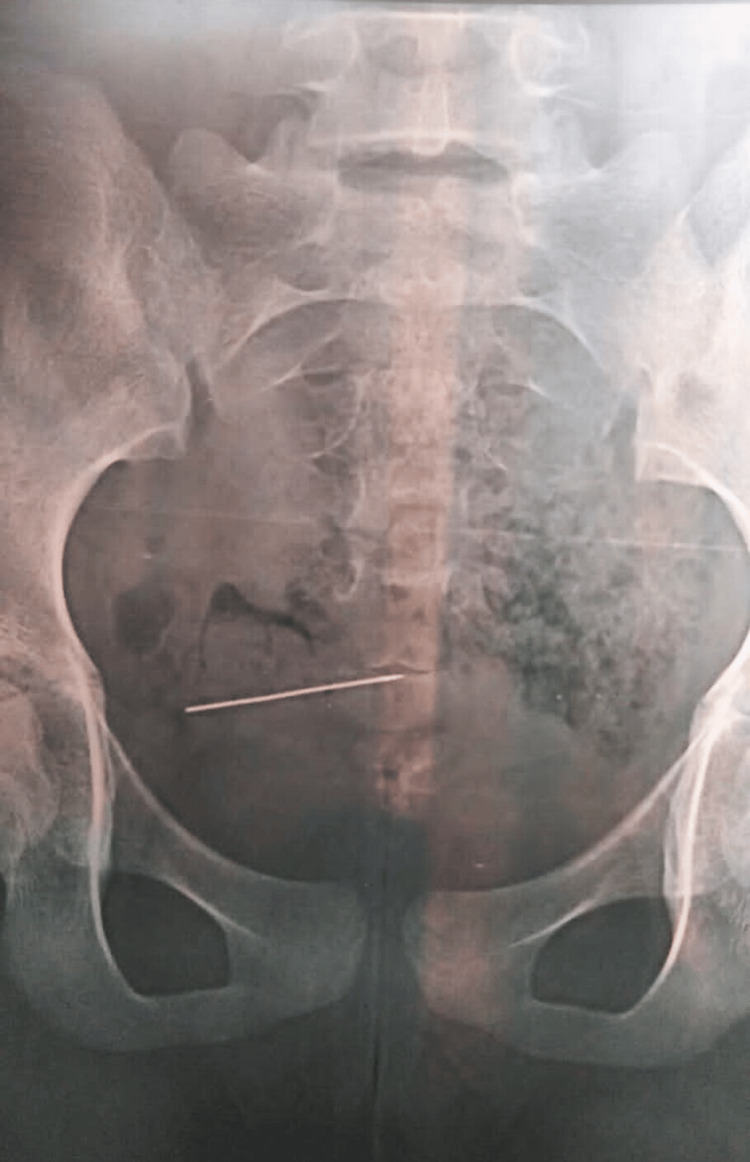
A pelvic radiograph in an anteroposterior view A straight needle is present in the pelvic cavity.
